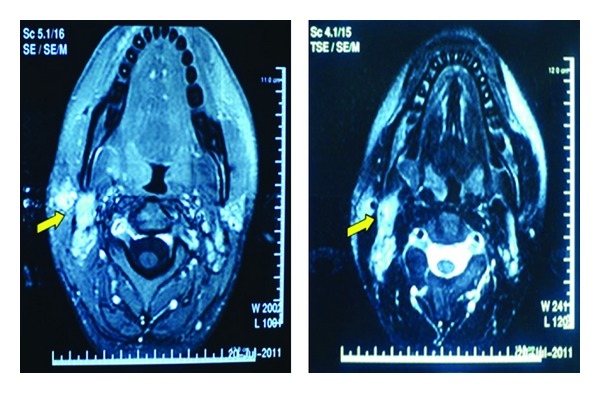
T1- and T2-weighted MR images showing right-sided cervical lymphadenopathy with focal hypointense areas with peripheral distribution and clear margins.
